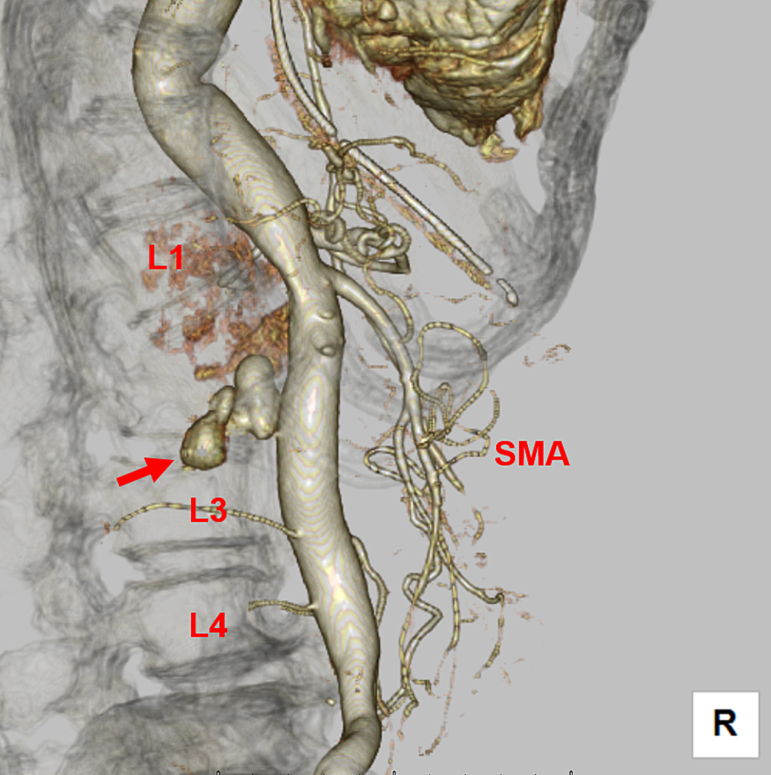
Three-dimensional computed tomography showing a pseudoaneurysm formed in the lumbar artery just below the aortic bifurcation (arrow). SMA: superior mesenteric artery.
